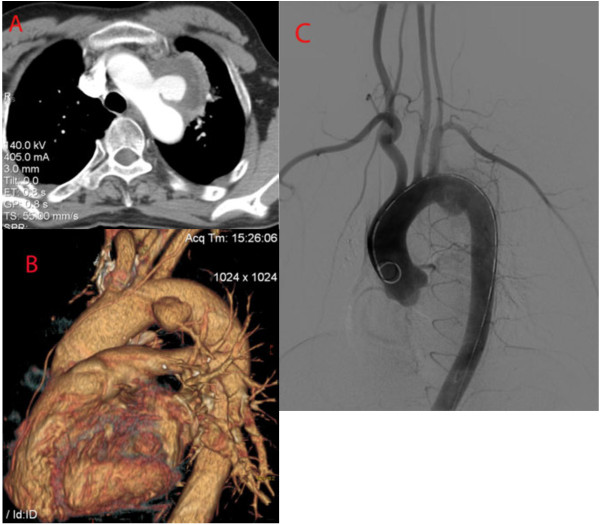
Preoperative CTA and arteriography: Thoracic CT scan showing an irregular wall at level of aortic arch (A,B). Preoperative arteriography demonstrated a pseudoaneurysm was evident between the origin of the left common carotid artery and the left subclavian artery; the left vertebral artery was dominant (C).
